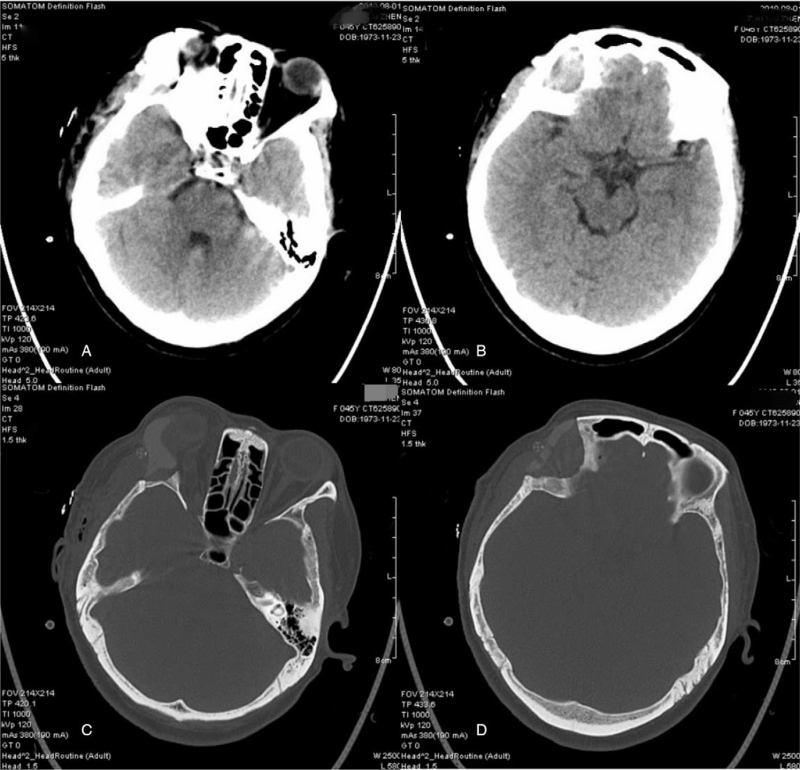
Postoperative CT scan showed a considerable degree of symmetry with PEEK prosthesis in case 2.
